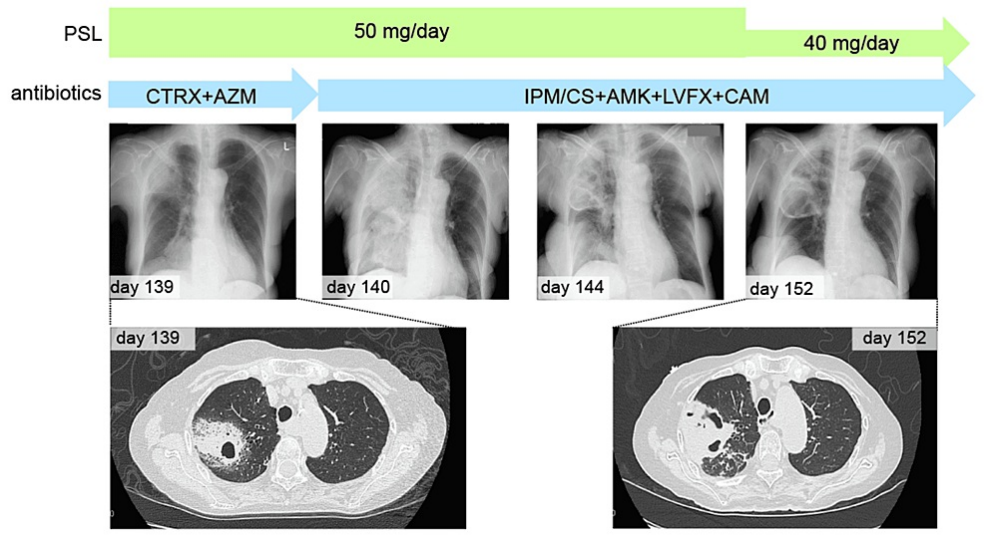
Chest X-ray and CT findings. Cavitary disease in the right lung persisted during antibiotic therapy. PSL, prednisolone; CTRX, ceftriaxone; AZM, azithromycin; IPM/CS, imipenem/cilastatin; AMK, amikacin; LVFX, levofloxacin; CAM, clarithromycin.
